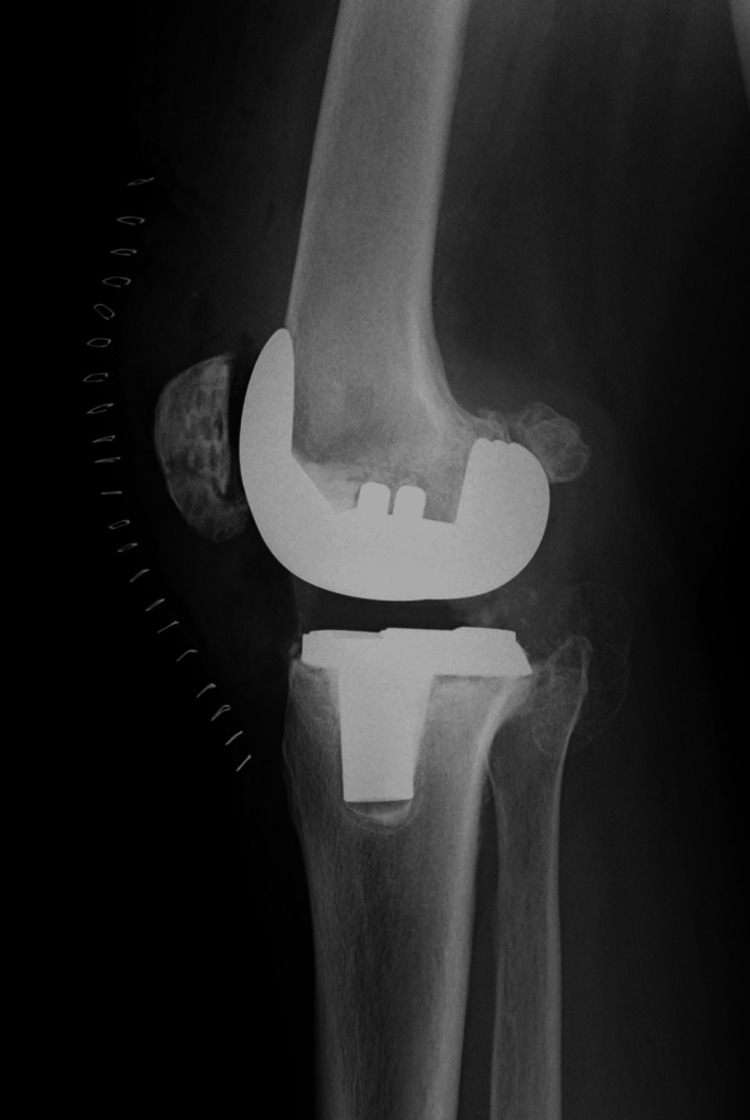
Postoperative X-ray after the initial surgery Osteosynthesis was performed with a Nesplon cable (Alfresa Pharma Corporation, Osaka, Japan). No loosening of the patellar component was observed during the operation and postoperative X-ray.
